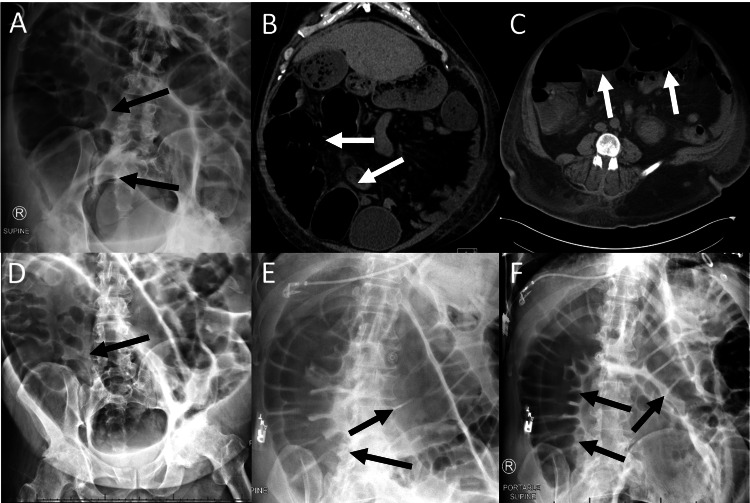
Imaging throughout the course of hospitalization. Initial plain film (A) showing cecal dilation to 12.6 cm. Coronal (B) and transverse (C) non-contrast computerized tomography images showing diffuse colonic and cecal dilation without evidence of mechanical obstruction. Plain film (D) three hours after the first neostigmine treatment showing a considerable reduction in colonic and cecal dilation to 6.2 cm. Plain film (E) 18 hours after the first neostigmine treatment showing recurrence of colonic and cecal dilation. Plain film (F) 20 hours after the second neostigmine treatment showing diffuse colonic and cecal dilation.
